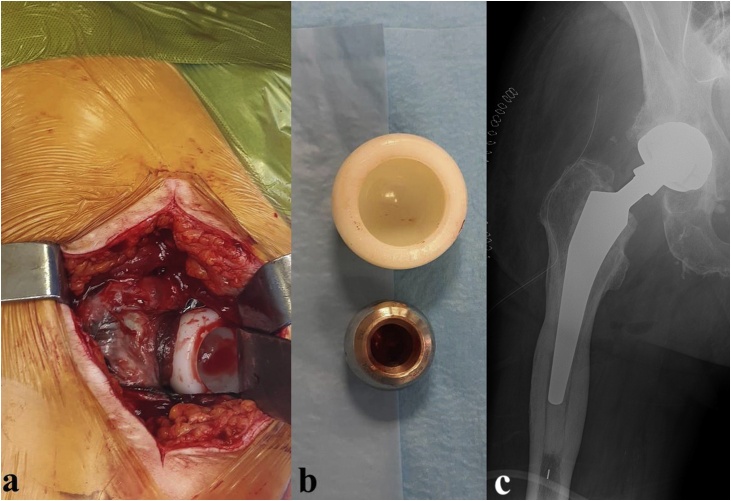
The PE liner was retrieved among soft tissues (a) with no noticeable sign of wear (b). Control radiograph after revision surgery (c).
